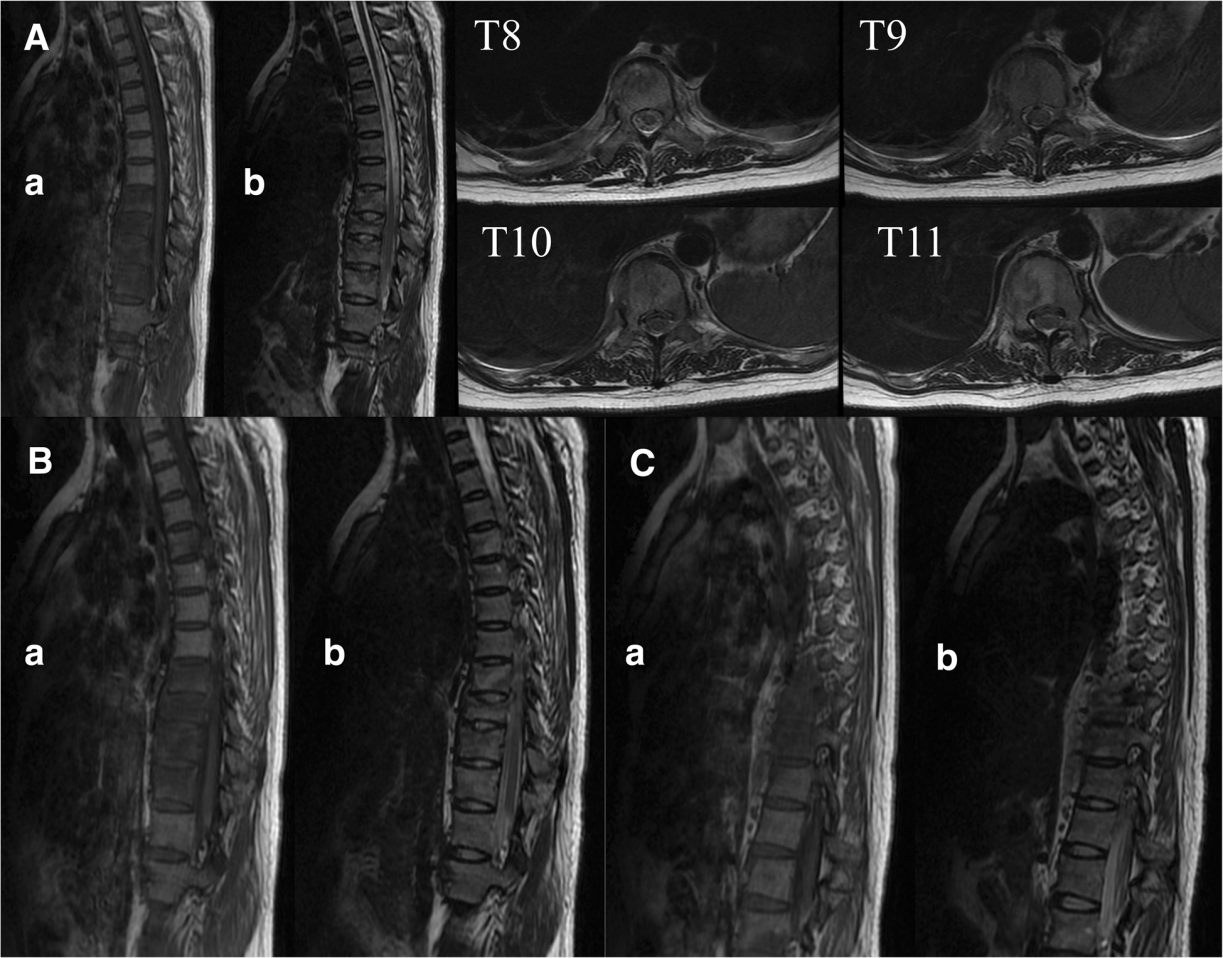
MRI scans of the thoracic showed superior end plate compression deformity in the T4 (b) and T8–11 vertebral bodies (a). In addition, diffused inflammatory bone changes were also noted in the T4 (b), T8–11(a) and L2 (c) vertebral bodies that were hypointense on the T1-weighted image (a) and hyperintense on the T2-weighted image (b)
